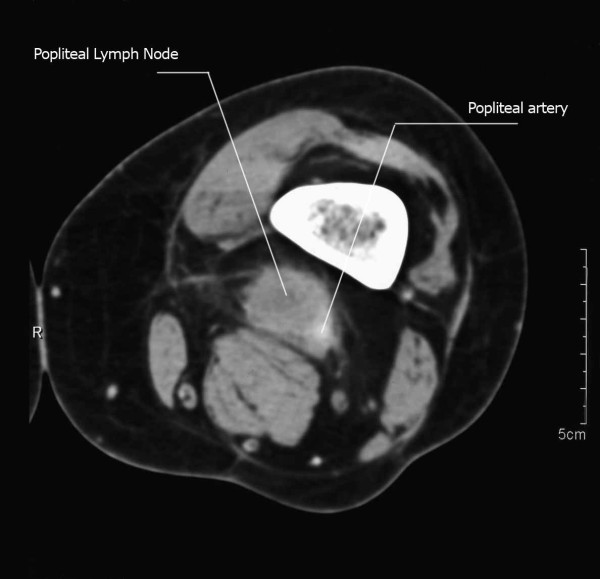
An MRI showing the popliteal metastasis.
